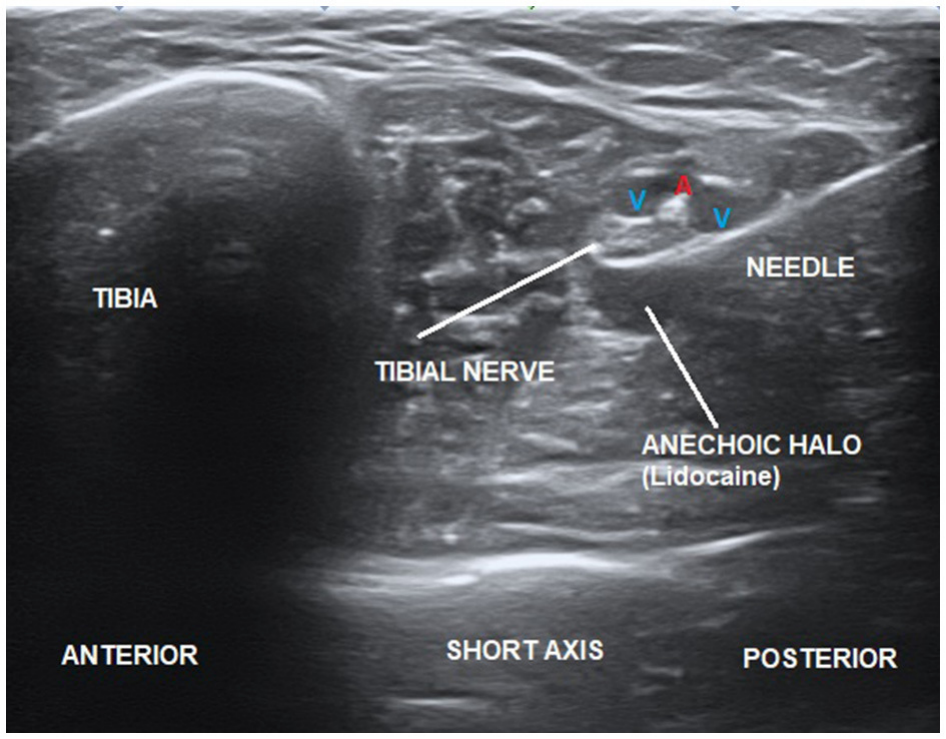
Ultrasound-guided injection of lidocaine close to the tibial nerve producing an anechoic halo.
